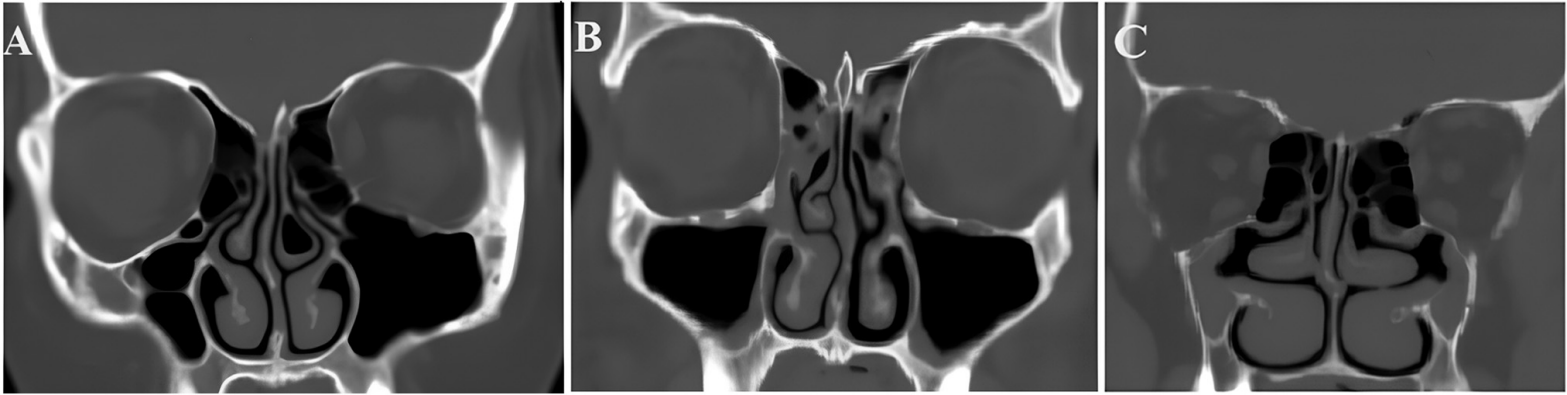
Computed tomography scans showing different directions of the UP: (
